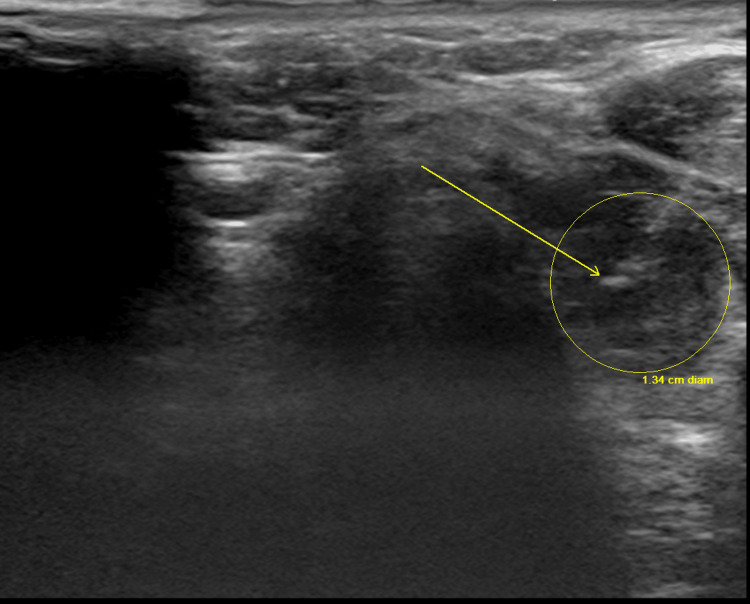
Demonstration of US-guided thyroid FNA biopsy, area marked in yellow FNA: fine needle aspiration; US: ultrasound
